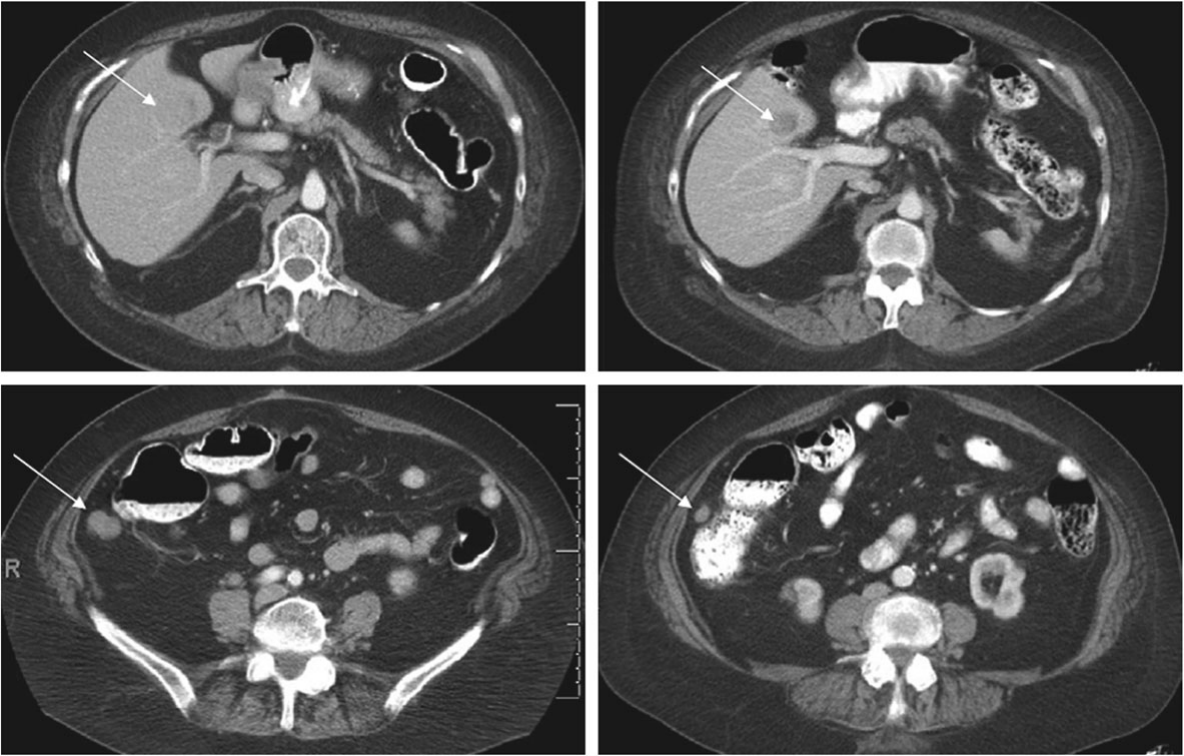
Tumor response with quizartinib monotherapy in a patient with GIST. Computed tomography scans of a patient with GIST demonstrated a 27% reduction in tumor burden with quizartinib monotherapy at the end of Cycle 1. Panels on the left represent baseline scans; panels on the right are from end of Cycle 1. *GIST* gastrointestinal stromal tumor
